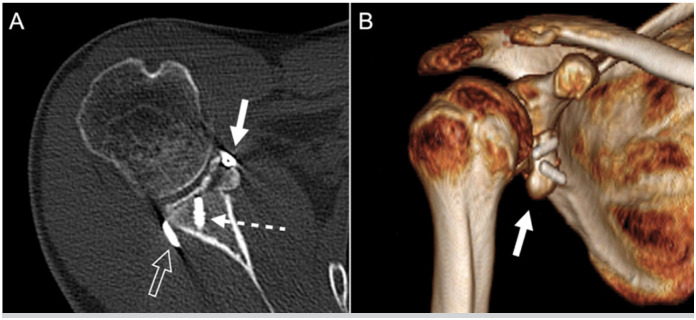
Right shoulder. Computed tomography at 24 months after surgery depicting adequate graft positioning (white arrow). A: Axial view; Hollow arrow points to the large posterior cortical button; Dashed arrow points to a metallic anchor used in the Bankart repair (previous failed surgery). B: Tridimensional reconstruction.
